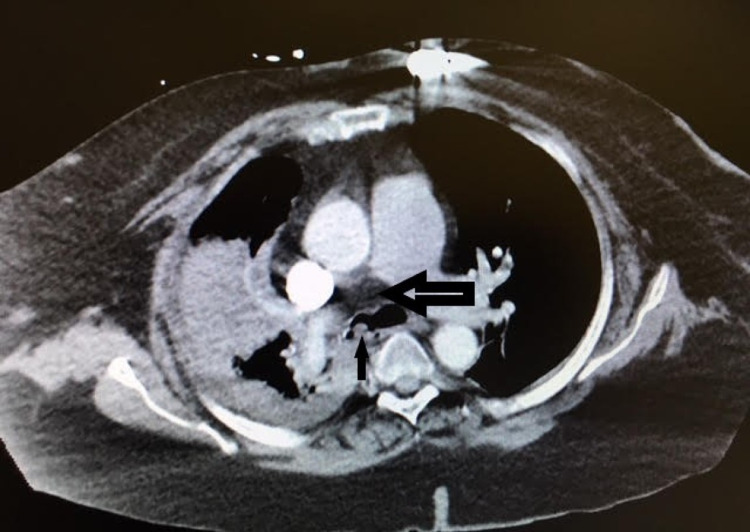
CT scan of the chest with a representative image showing density in right mainstem bronchus (solid black arrow), while the black and white (right) arrow indicates pre-carinal lymph node CT: computed tomography
